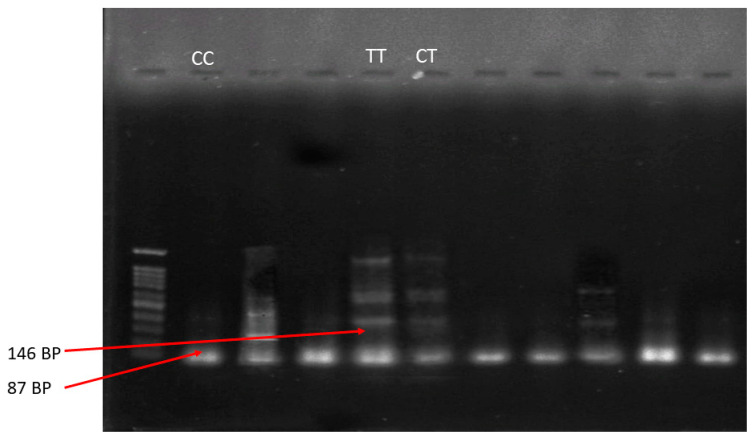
Agarose gel electropherogram of MTHFR (rs1801133), or genotype (CC, TT, and CT) performed by Tetra-ARMS PCR.
